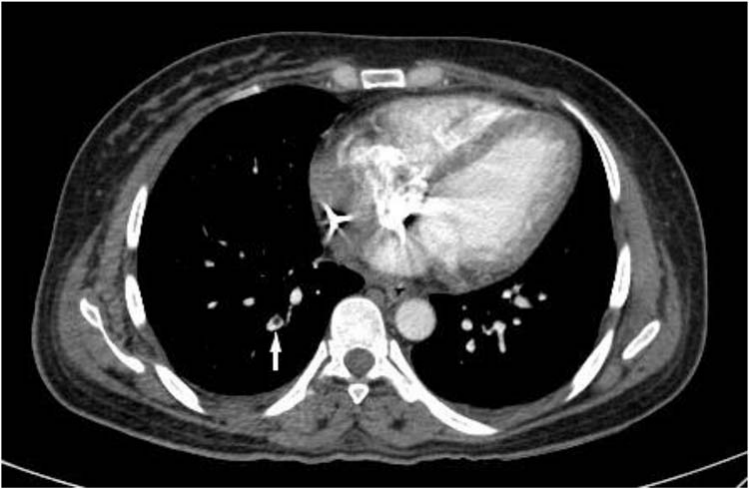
CT pulmonary angiogram on the third day of hospital admission shows a filling defect in a subsegmental branch of the right posterior pulmonary artery (white arrow), compatible with a PE.
